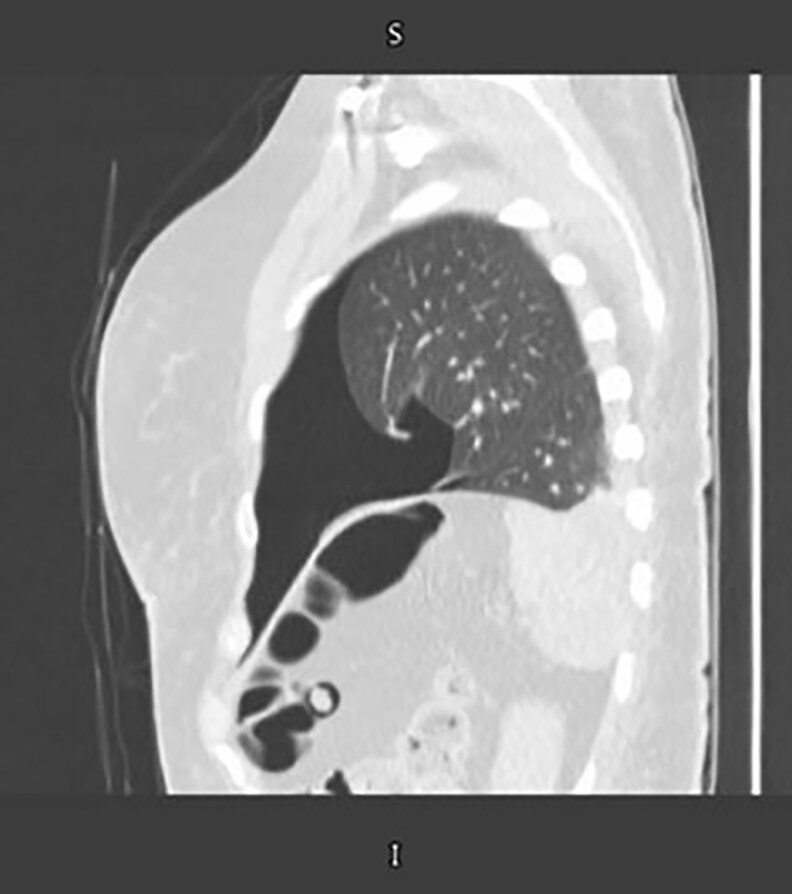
Computed tomography scan sagittal view showing a collapsed left lung in a 48-year-old female patient, confirming moderate pneumothorax.
